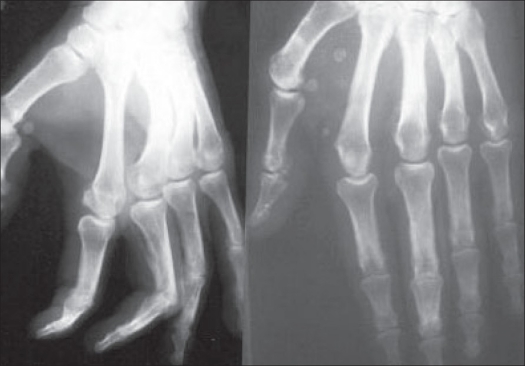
Radiographs after Kirschner wire removal showing union of fracture and good alignment
